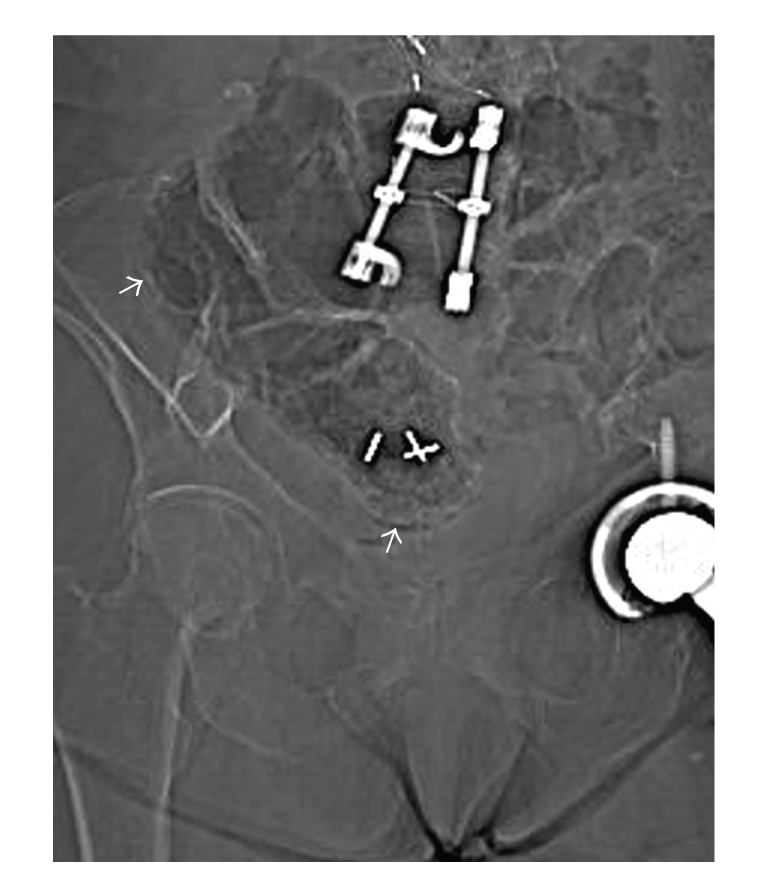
Scout film from abdominal and pelvic CT scan shows crecentic lucencies in the walls of the stool-filled cecum and ascending colon, compatible with pneumatosis.
